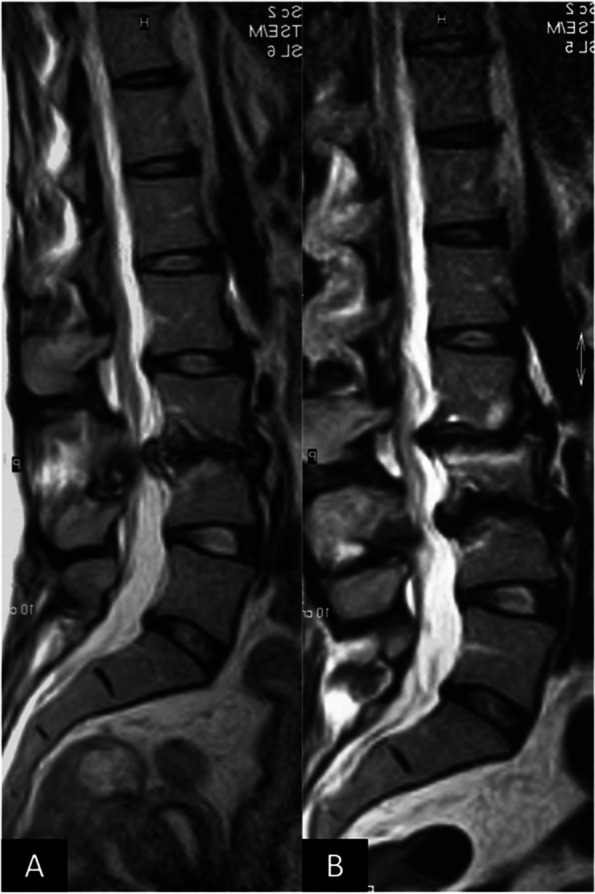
A and B: comparison of T2-weighted MRI scans from September 2003 (A) and April 2007 (B). Figure B shows destruction and osteophyte formation of the L3/4 disk space, the first one involved in the pathologic process, and bone edema in L2/3, which appeared healthy in the previous imaging (Figure A)
